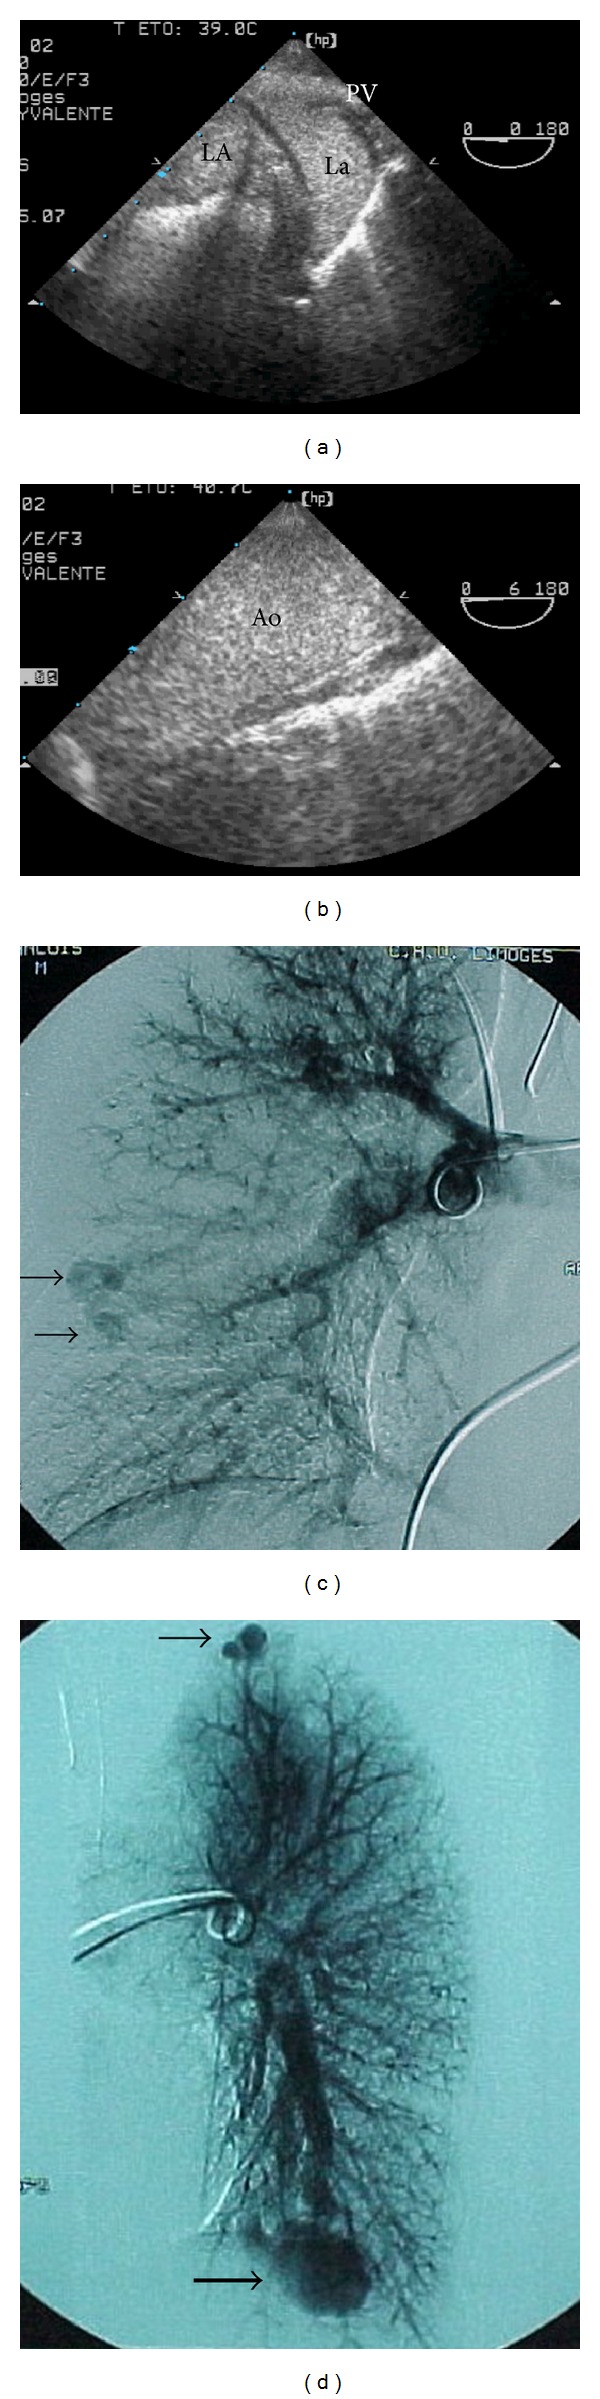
Pulmonary arteriovenous fistulae associated with the Rendu-Osler-Weber disease. The contrast study performed during a transesophageal echocardiographic examination depicted a massive opacification of the left atrium a few beats after the opacification of the right atrium through the left and right pulmonary veins (a), but no patent foramen ovale, and a subsequent massive opacification of the aortic arch (b). Pulmonary angiography confirmed the presence of multiple arteriovenous fistulae in the two lungs ((c) and (d), arrows), the largest being located in the left inferior lobe (thick arrow). These lesions were subsequently excluded from the pulmonary circulation by serial percutaneous transcatheter embolizations. LA: left atrium; La: left atrial appendage; PV: pulmonary vein; Ao: aortic arch.
